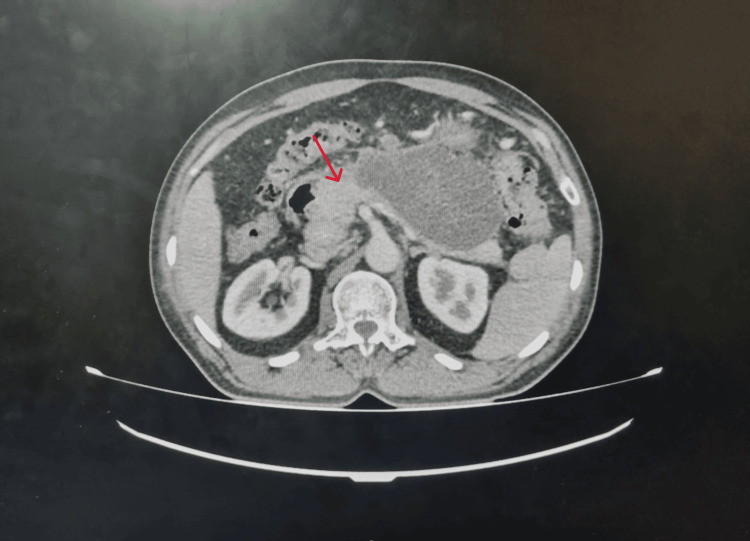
Contrast-enhanced computed tomography (CECT) of the abdomen showing an enlarged pancreas with peripancreatic inflammation and areas of necrosis (indicated by a red arrow), consistent with acute necrotizing pancreatitis.
